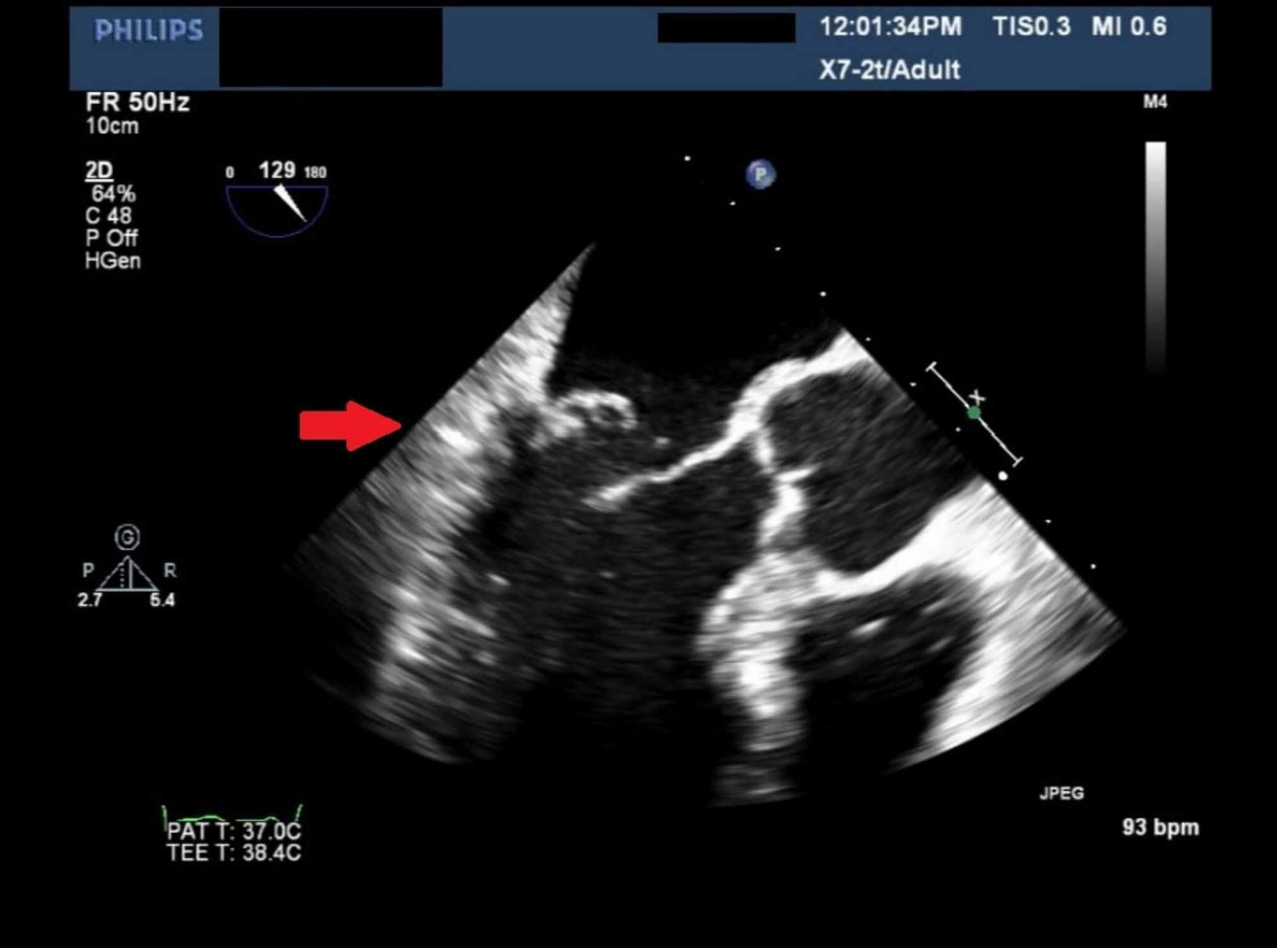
Two-dimensional echocardiography Anteriorly directed severe mitral regurgitation. Absent aortic valve disease.
